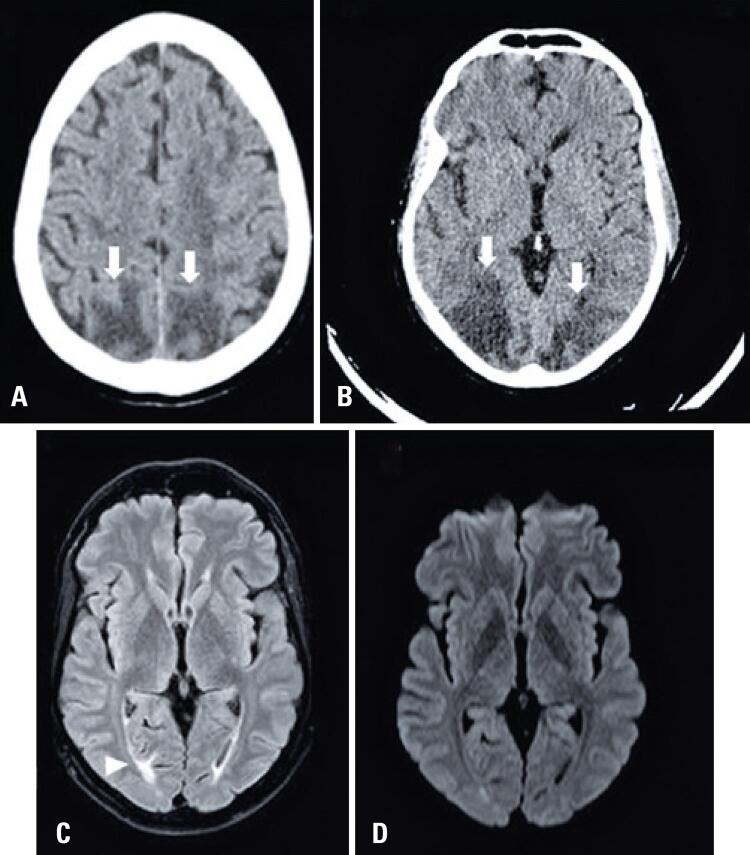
Patient 10. Computed tomography scan. (A and B) Axial sections show hypodensity in the subcortical white matter of the parietal and occipital lobes (white arrows). (C) Axial fluid-attenuated inversion recovery images: hyperintense area in the left parieto-occipital white matter (white arrowhead), with reduced dimensions in comparison to the lesion area seen on the previous computed tomography scan. Diffusion-weighted (D) images in the axial plane. There were no diffusion restriction areas
